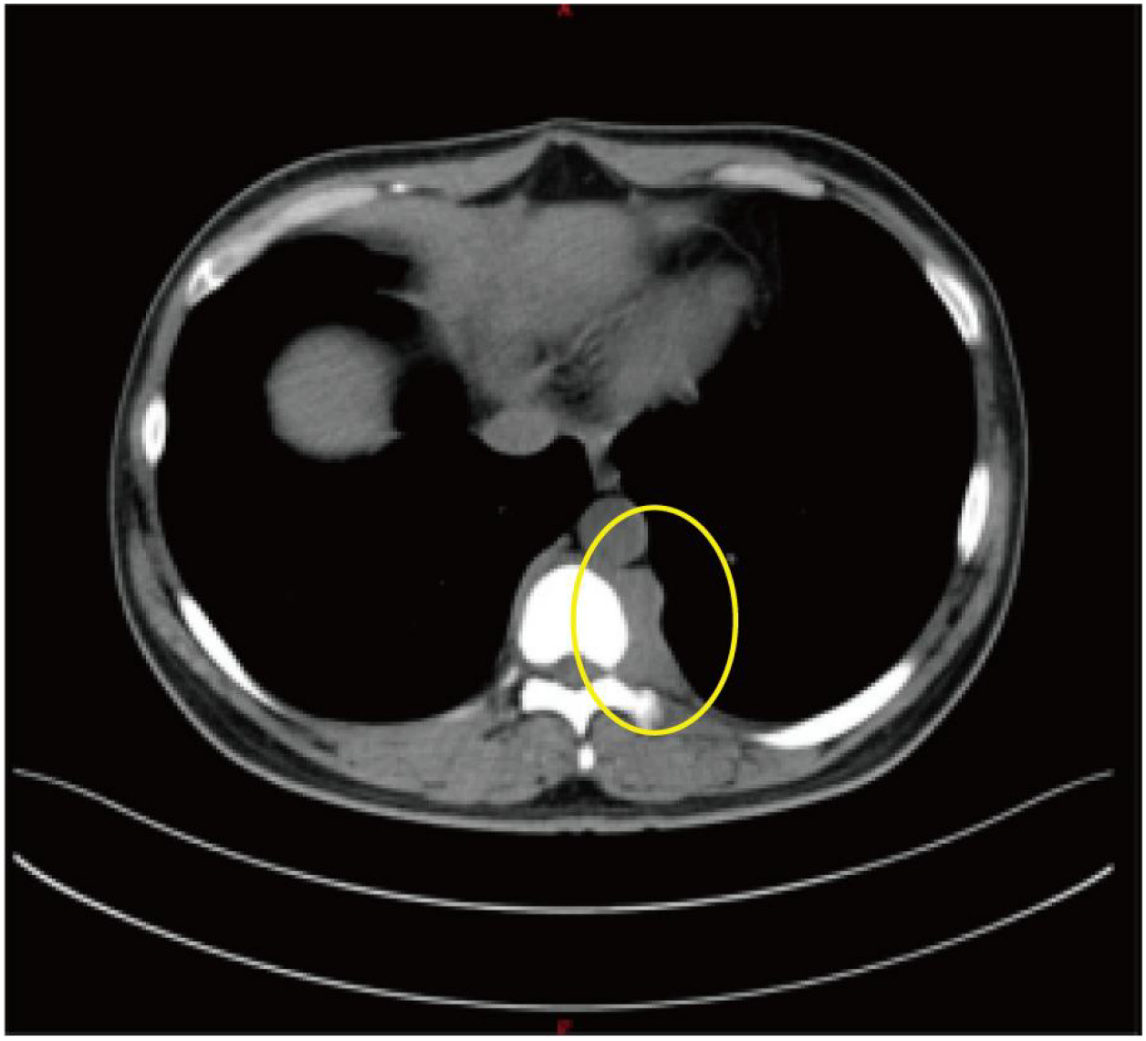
Axial chest CT revealing paravertebral and mediastinal mass (The yellow circles indicate the location of the myeloid sarcoma.).
